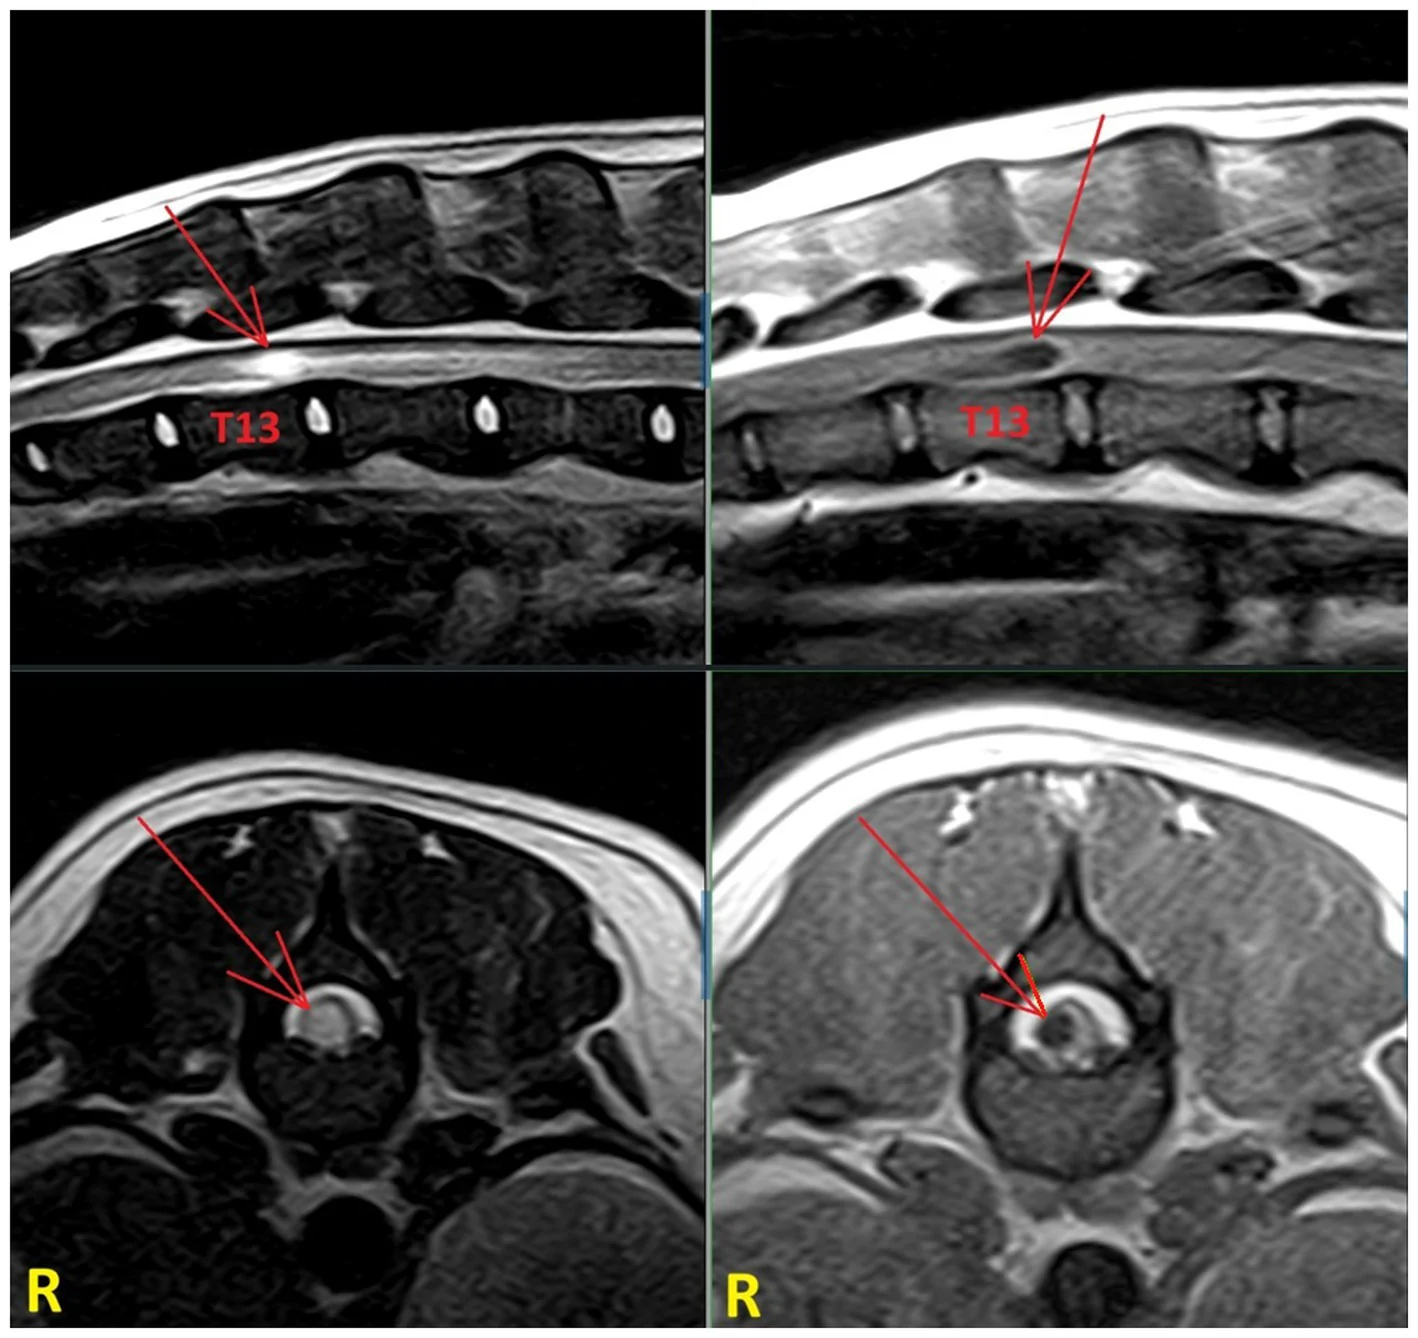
Upper left and upper right: T2W and T1W sagittal MRI images. Lower left and lower right: T2W and T1W + C transverse MRI images: A teardrop cystic structure in the spinal cord over the T13 vertebral body (red arrows).
